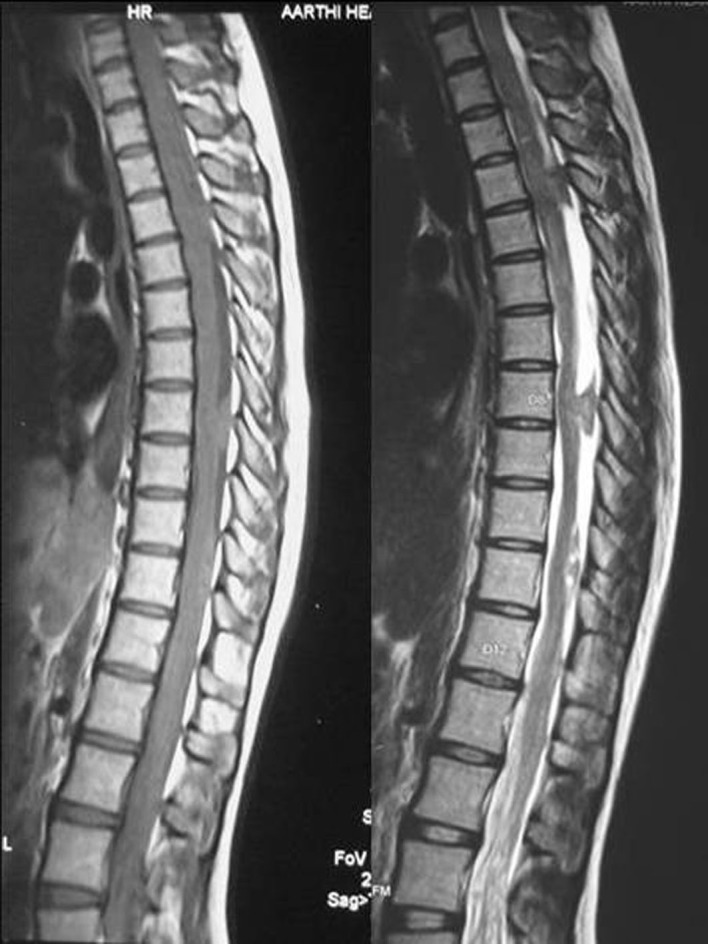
MRI scans of the spine (sagittal view) showing multiple intradural extramedullary lesions at D3-D4, D8 and D10-D11 which were isointense on T1W and hyperintense on T2W.
